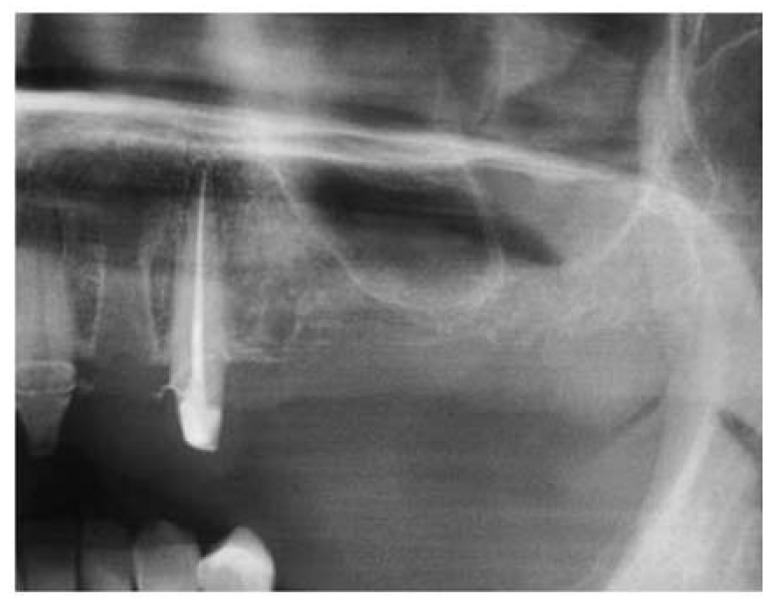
Preoperative panoramic X-ray view.
